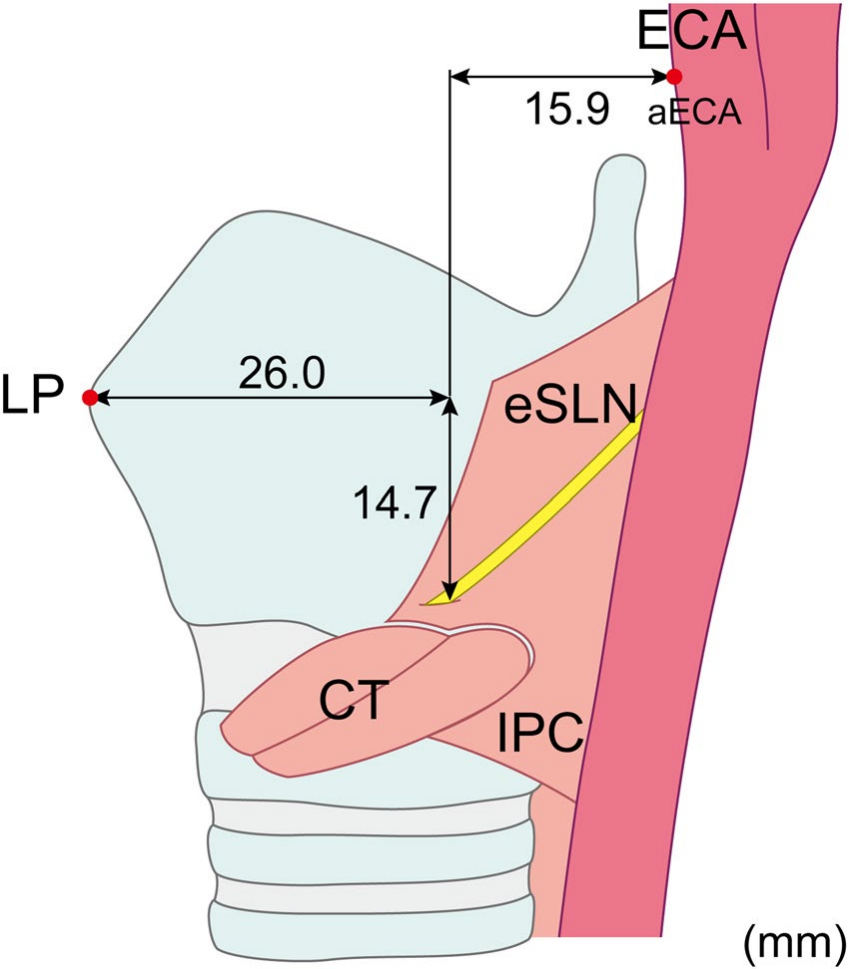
Location of the piercing point of the external branch of the superior laryngeal nerve (eSLN) with reference to the laryngeal prominence (LP) and the anterior border (aECA) of the external carotid artery (ECA). IPC, inferior pharyngeal constrictor; CT, cricothyroid.
